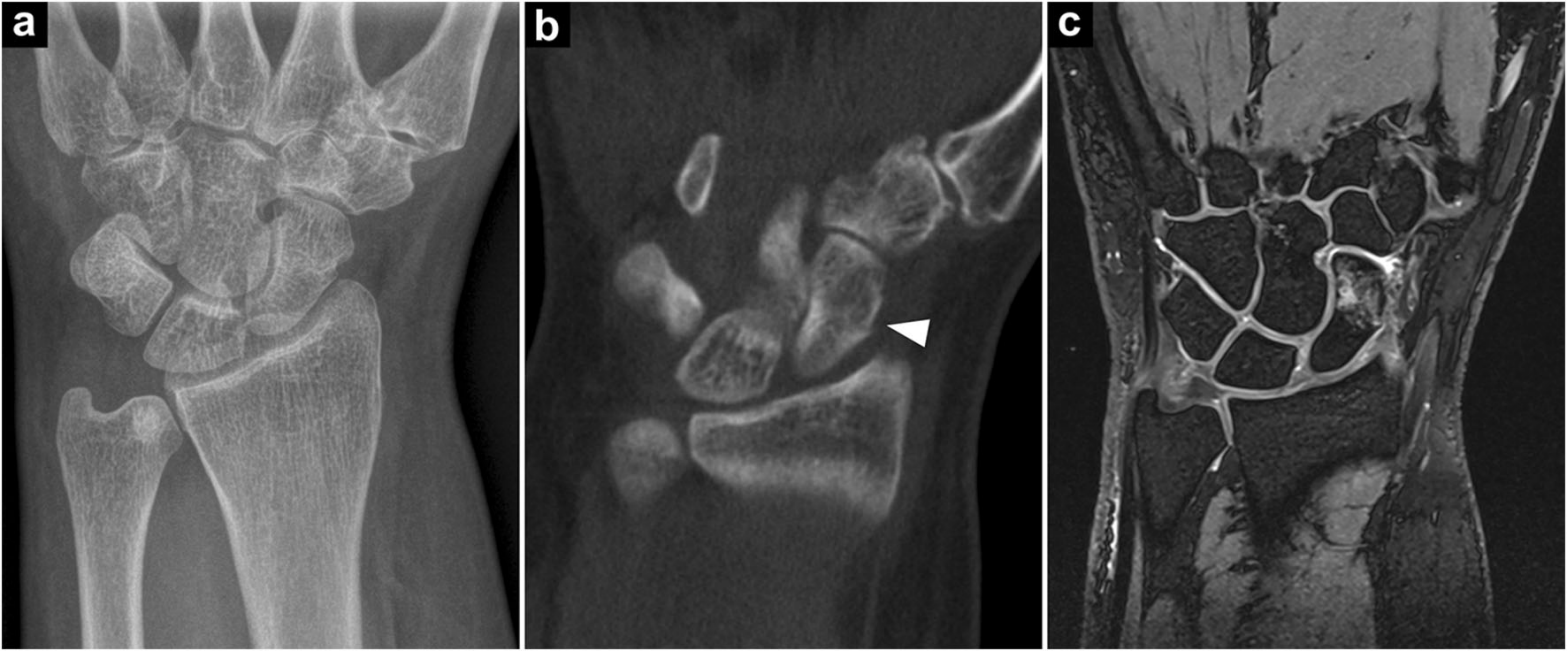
A 55-year-old woman presented to the emergency department with radial-sided left wrist pain and swelling after slipping during yoga class. Initial radiography was deemed negative for fracture presence (a). Subsequent cone-beam CT demonstrated a subtle fracture line in the waist of the scaphoid bone (arrowhead), corresponding to a potentially unstable B2 fracture (b). Suggested treatment was upgraded to surgery; however, the patient decided for conservative therapy. MRI follow-up after 1 week confirmed the fracture diagnosis, depicting extensive bone bruise in the distal portion of the scaphoid (c)
